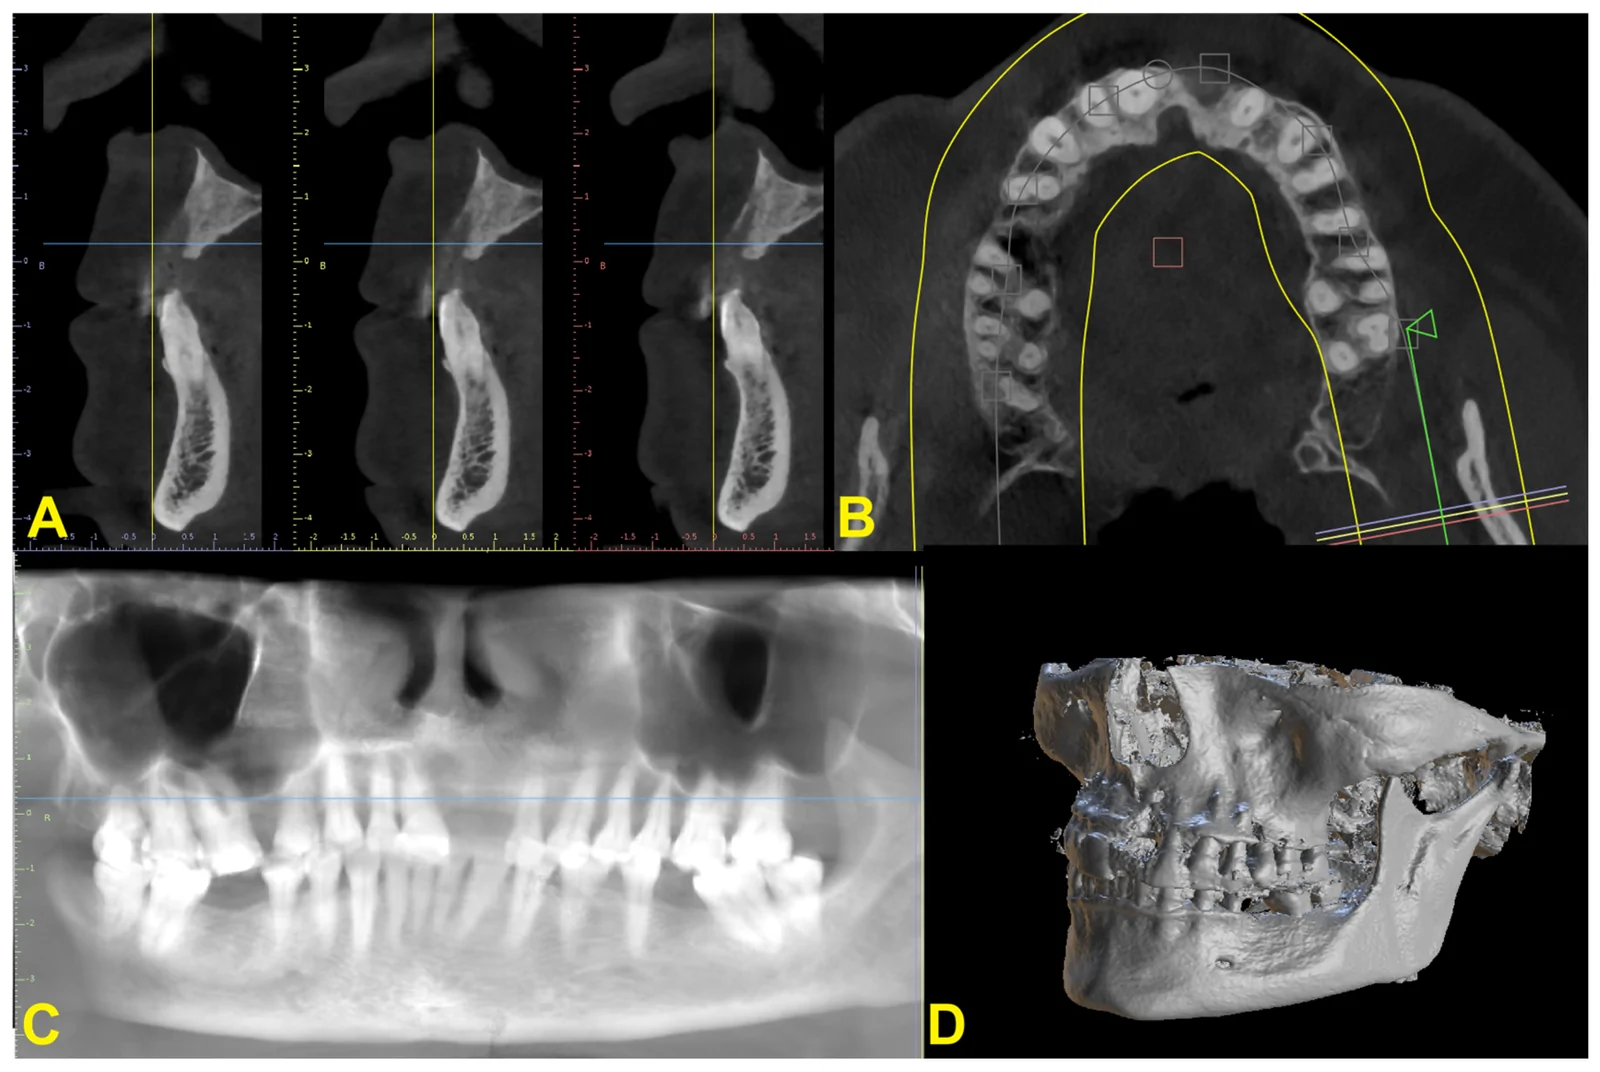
Cone-beam computed tomography of tooth 21 region four months after initial implant procedure: (A) cross-sectional views; (B) axial view; (C) panoramic reconstruction; (D) pseudo-3D reconstruction.
